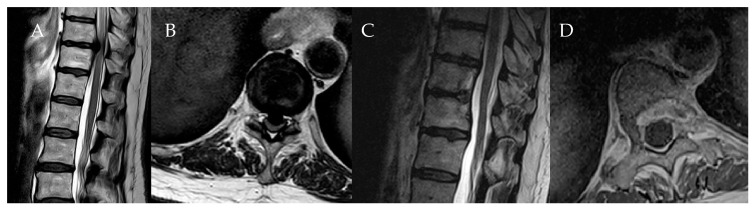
Patient No. 5. Preoperative T2-weighted (A) sagittal and (B) axial MRI with postoperative T2-weighted (C) sagittal and (D) T1-weighted post-contrast axial MRI of the thoracic spine, which shows the complete resection of the herniated disc.
